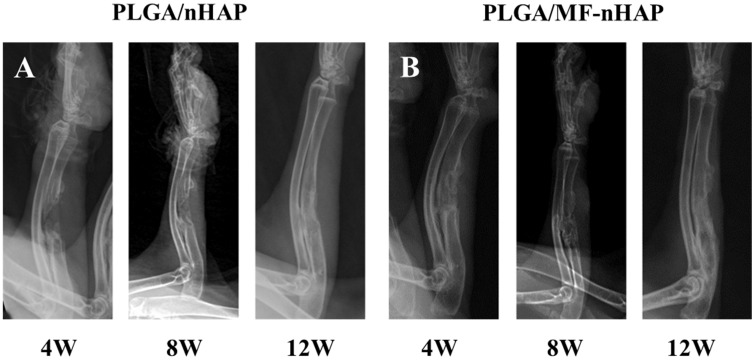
X-ray examination of (A) PLGA/nHAP and (B) PLGA/MF-nHAP scaffolds implanted in ulnas defect at different periods.
